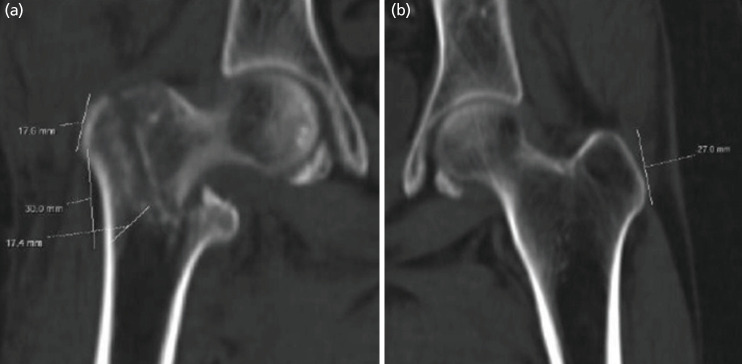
(a, b) AO Type A23 case: measurement via 2D CT scan where measured lateral wall thickness (17.4mm), ipsilateral greater trochanteric height 17.6mm, contralateral greater trochanteric height (27mm).
